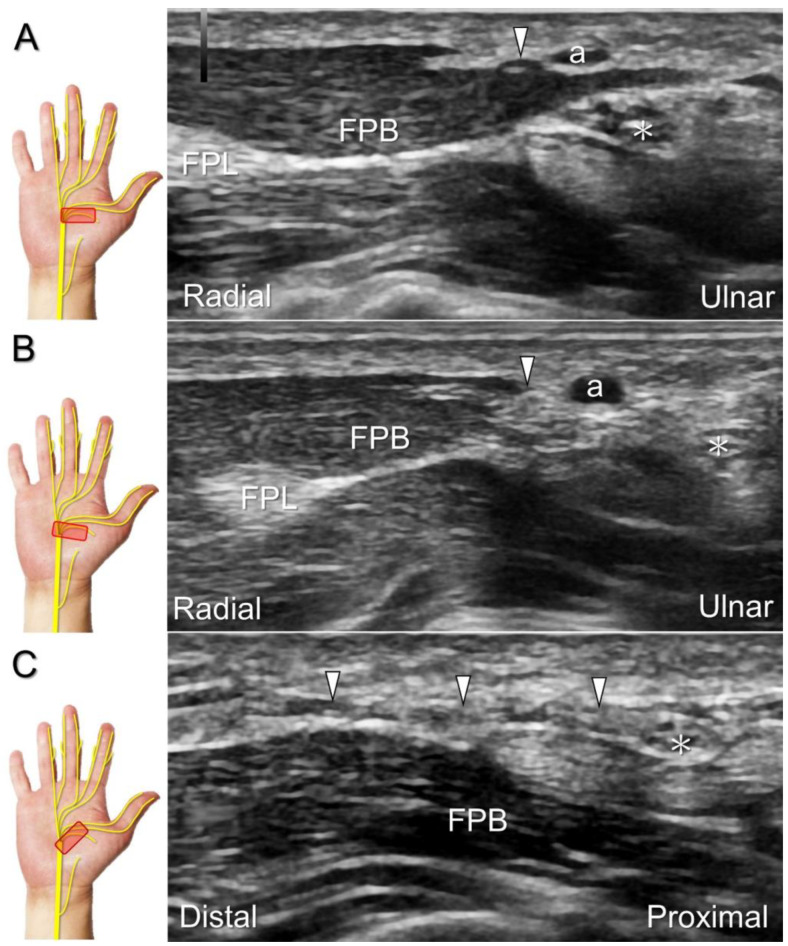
Sonographic imaging for the recurrent motor branch of the median nerve. Tracking back from the surface of the thenar muscle (A) toward the division site from the main trunk in short-axis (B) and long-axis (C) imaging. Asterisk: median nerve; white arrowheads: recurrent motor branch of the median nerve; a: artery; FPL: flexor pollicis longus tendon; FPB: flexor pollicis brevis muscle.
